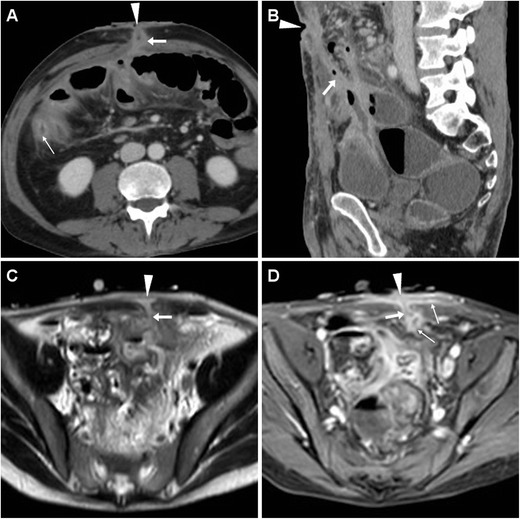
Two cases of post-surgical ECF in CD. After recent ileocecal resection, in a 61-year-old man multiplanar CT-enterography (A, B) showed mural thickening with mucosal hyperenhancement (thin arrows) along distal ileum consistent with disease activity, and an ECF (arrows) coursing obliquely from the affected tract to small-sized mesogastric EFO (arrowheads); reoperation included ECF debridement, ileal resection and redo anastomosis. A 27-year-old man developed an ECF after subtotal colectomy with cecal-rectum anastomosis, with corresponding MRI (T2-weighted image C, fat-suppressed post-contrast T1-weighted image D) appearance of fluid-filled track (arrows) with peripheral enhancement (thin arrows). Prominent inflammation also affected the skin surrounding the EFO (arrowheads) and at inner aspect of the ECF: the latter corresponded endoscopically to the anastomotic site and was treated by positioning of an over-the-scope clip, allowing ECF resolution
